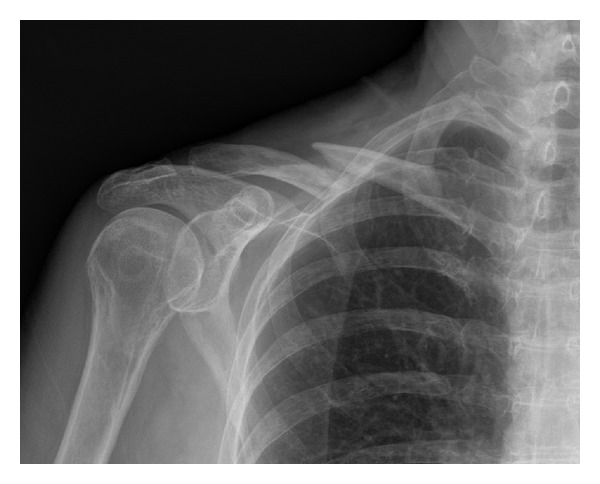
Radiograph from date of injury showing the right mid-shaft clavicle fracture. A 2.5 cm fragment is displaced posteriorly, but is not seen well in this radiograph.
